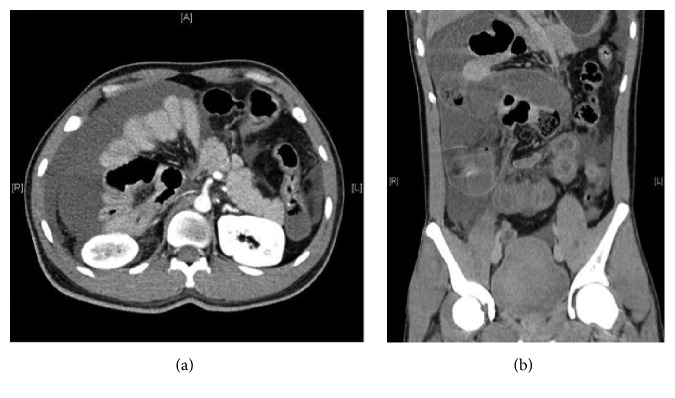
Contrast-enhanced CT in (a) axial and (b) coronal sections showing clustering of dilated small bowel loops in the right side of the abdomen, with no visualization of an encapsulating sac. The superior mesenteric artery is to the right of the superior mesenteric vein (a).
